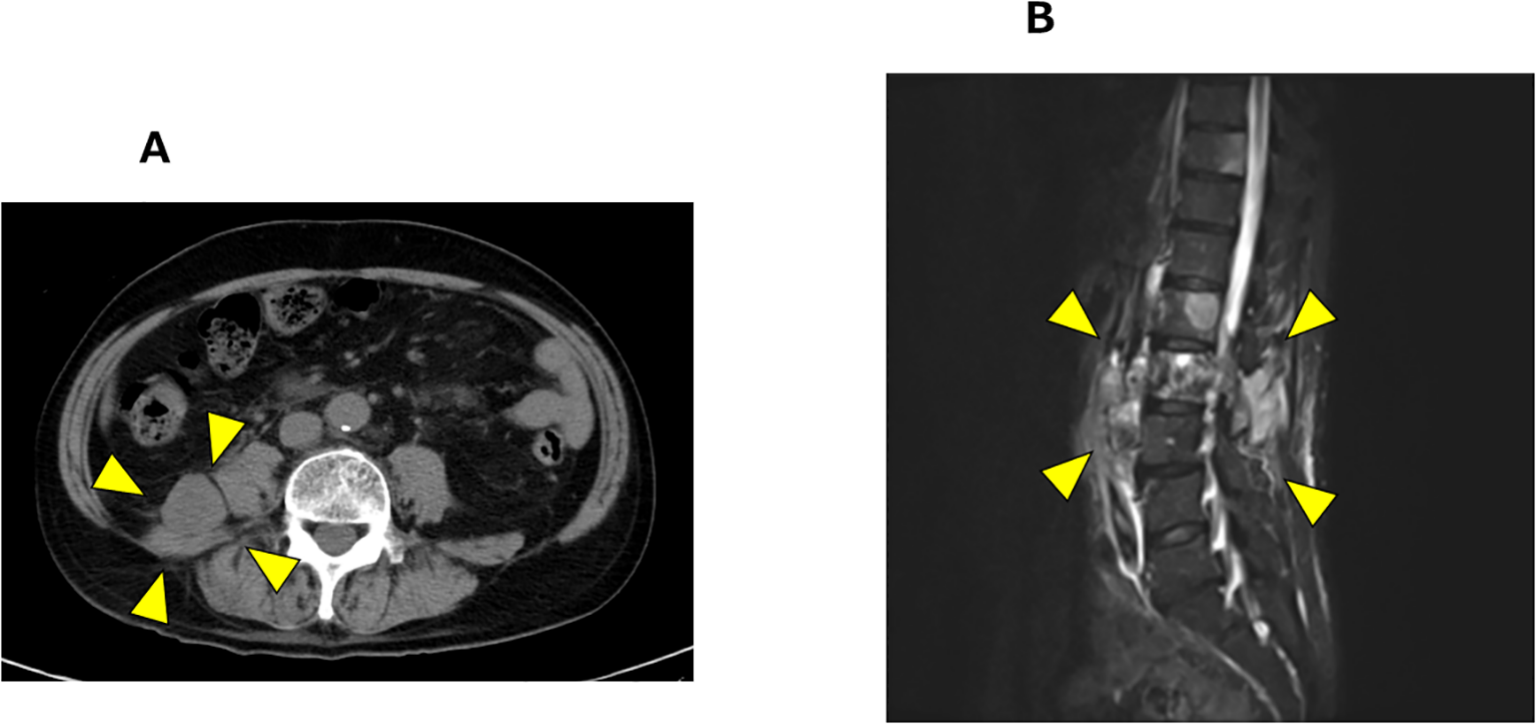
Radiological progression after abiraterone with prednisolone therapy. (A) Pelvic CT showing retroperitoneal mass (arrow heads). (B) T1 weighted MRI revealing progression of lumbar vertebral metastases (arrow heads).
